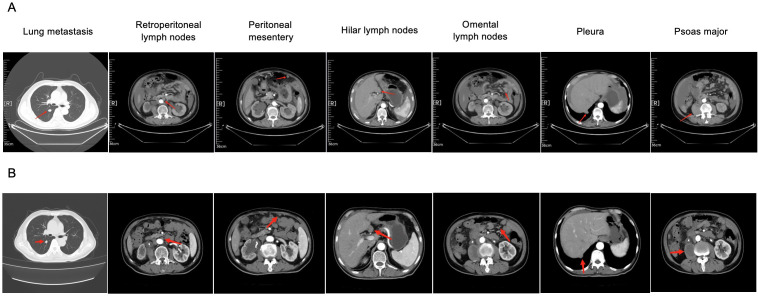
CT scans of the chest and abdomen before (A) and after (B) the treatment guided by the organoid drug screening. Red arrows head towards to the lesions in different locations.
